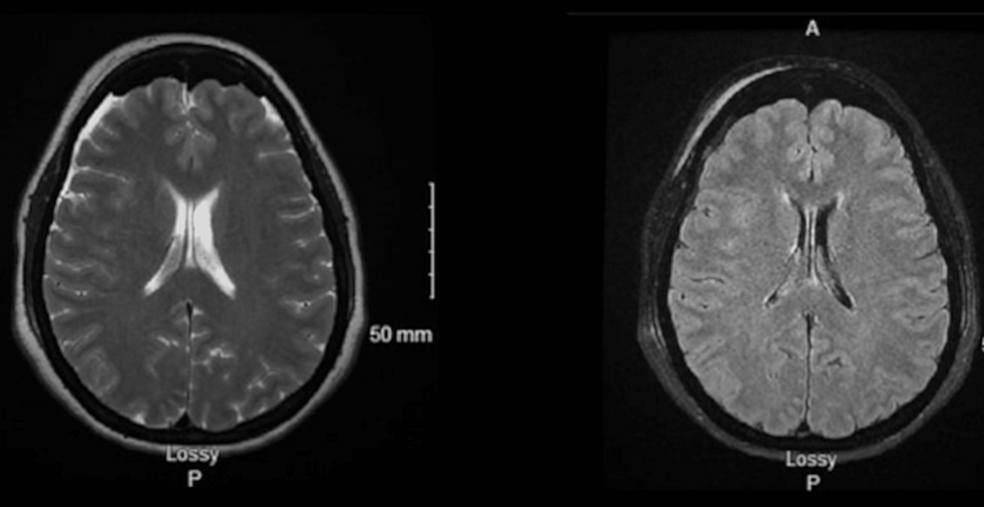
Axial magnetic resonance imaging of the brain. Normal brain parenchyma and ventricles without hydrocephalus or mass lesions.
